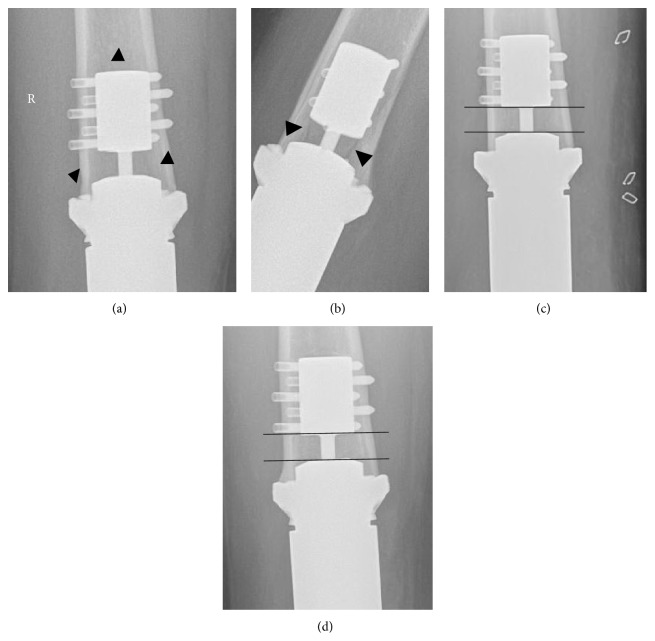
(a) and (b) Radiolucent line in the compress area after falling 1 y postop, (c) and (d) development of angulation and loss of distance between the anchor plug and the spindle can be observed after fracture (d) healing when compared to the prefracture X-ray (c). At 18 months stage 3 has not been reached (d).
